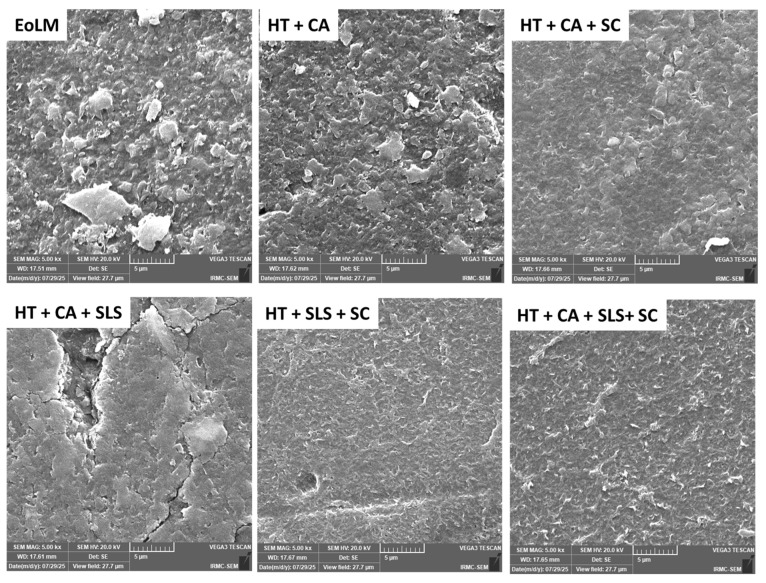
SEM images of different RDEoLM using different chemo-ultrasonic cleaning methods.
